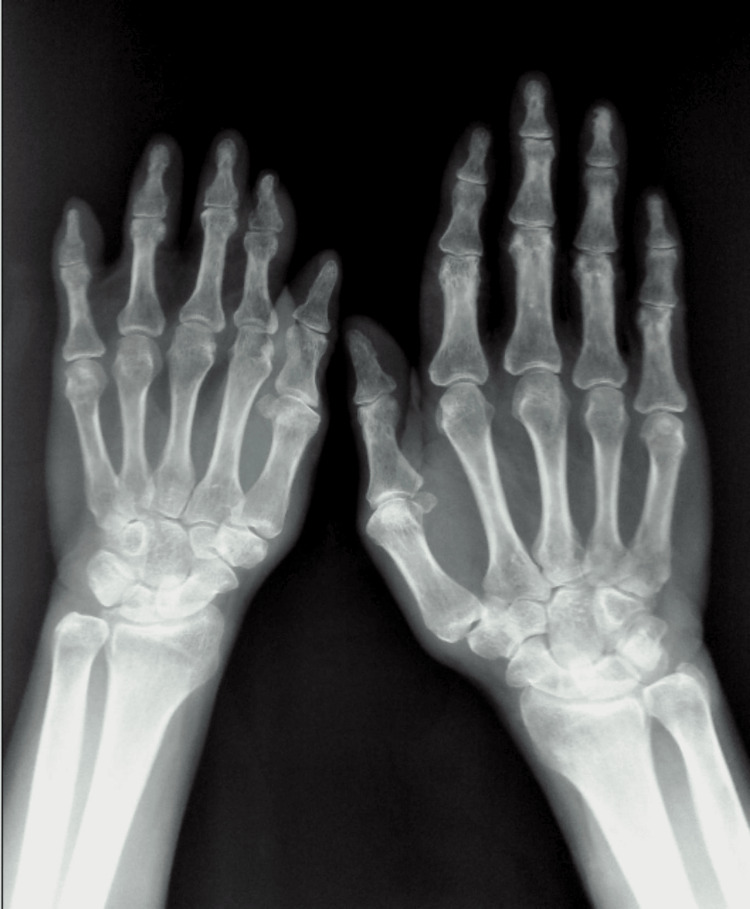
X-ray of hands showing hypoplasia of middle and distal phalanges of index, ring, middle, and little fingers of the right hand, with a normal left hand.
